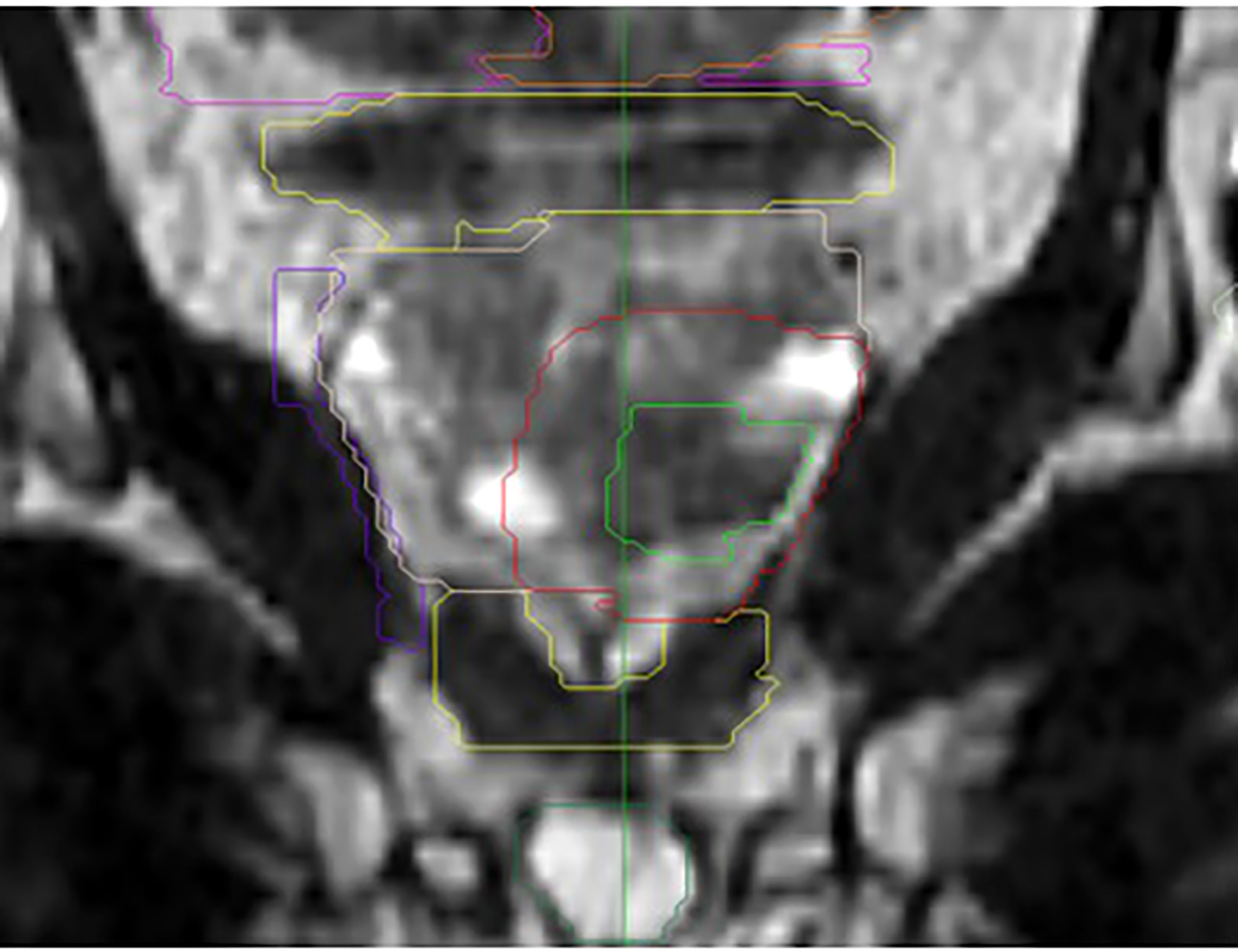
Example of delineated focused stereotactic treatment on the MRI T2 sequence frontal view. Purple = contralateral neurovascular bundle. Lower yellow structure = external sphincter. Light green = gross target volume. Red = clinical target volume. Dark green = penile bulb.
